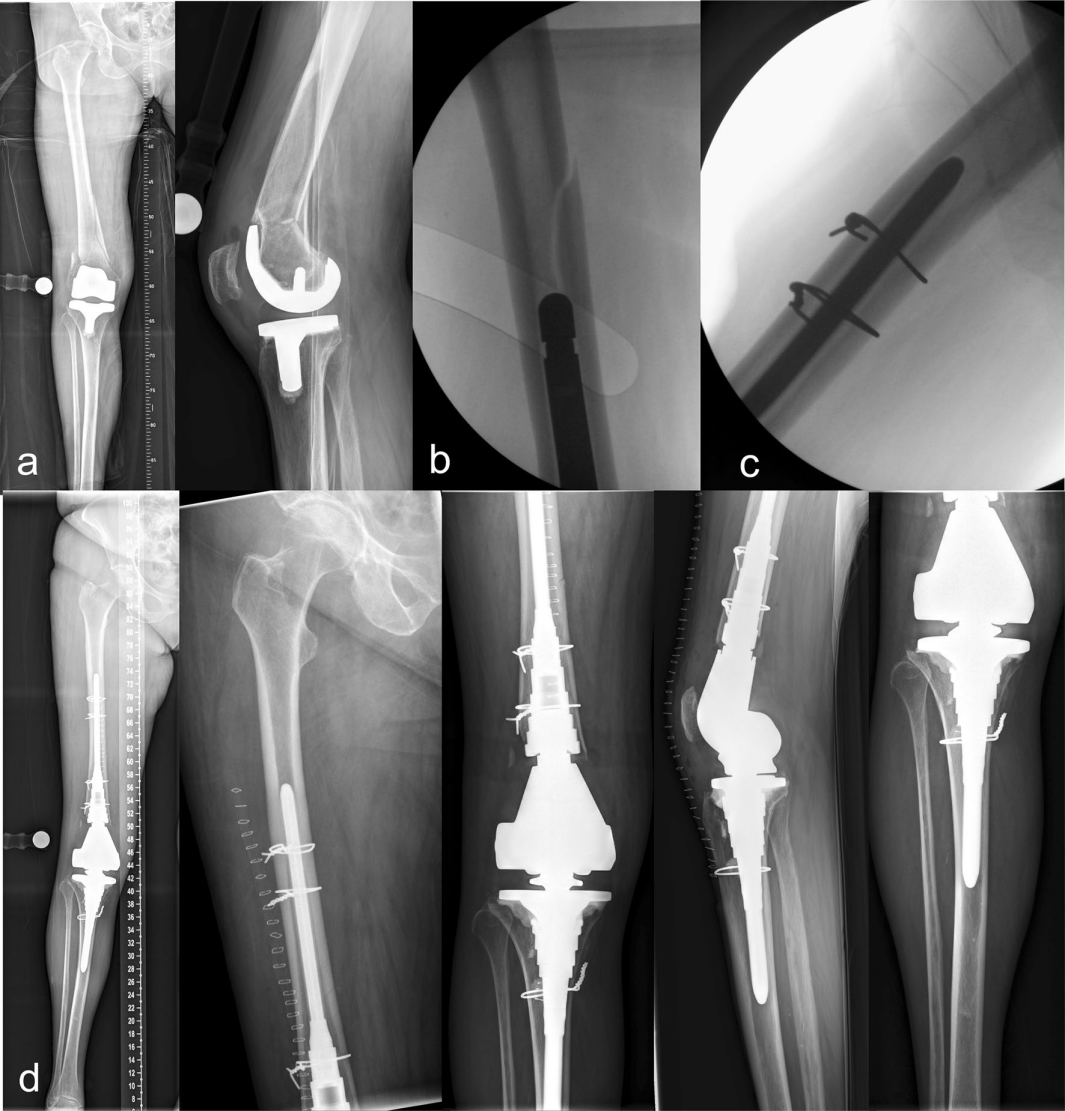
X-rays of an intraoperative periprosthetic fracture of the proximal tibia and proximal femur in a distal femoral replacement (a), known by clinical findings and intraoperative x-ray (b), which was treated with the implantation of a longer stem and cerclage (c) as well as partial weight bearing for six weeks postoperatively (d).
